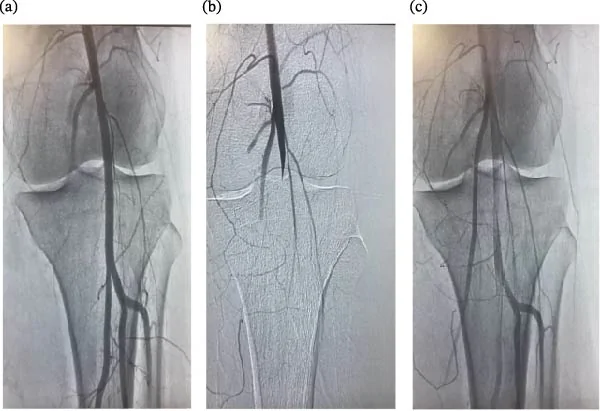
Dynamic CT angiography of the left lower limb. (a) Normal popliteal artery flow during plantar flexion. (b) Arterial occlusion during foot dorsiflexion. (c) Restoration of blood flow after release of dorsiflexion.
